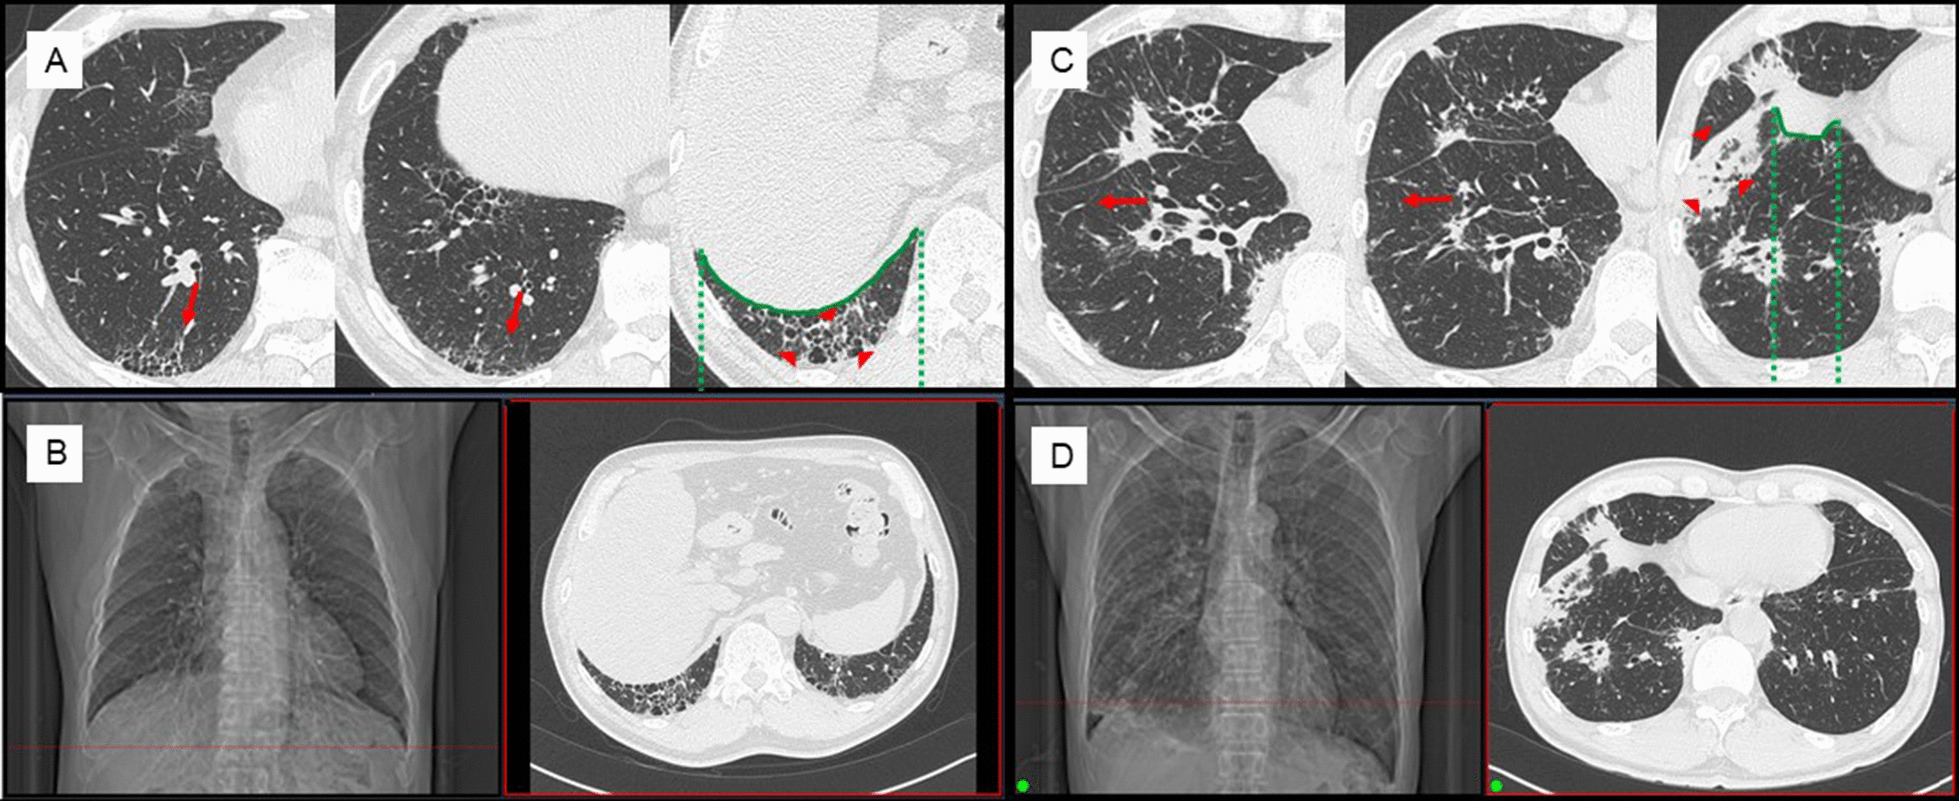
Chest HRCT findings of diaphragm overlap sign. a Case positive for diaphragm overlap sign. Right B^10^ b + c branched into a subsegmental bronchus (arrows); the peripheral lesion included reticular shadows and ground-glass opacities with traction bronchiectasis (arrowheads). In the anterior–posterior direction, this S^10^b + c area and the diaphragm completely overlapped (inside of the green area) and were judged to be positive for diaphragm overlap sign. b The S^10^b + c lesion is located at the red line in the CT scout view, and the coronal section shows that it is completely subdiaphragmatic in height. c Case negative for diaphragm overlap sign. Right B^8^ branched into a subsegmental bronchus, and then B^8^a proceeded laterally (arrows); the peripheral lesion of B^8^b included a consolidation (arrowheads). In the anterior–posterior direction, S^8^b did not overlap with the diaphragm (outside of the green area) and was judged to be negative for diaphragm overlap sign. d The S^8^b lesion is located at the red line in the CT scout view, and the coronal section shows that it to be at a height above the diaphragm
